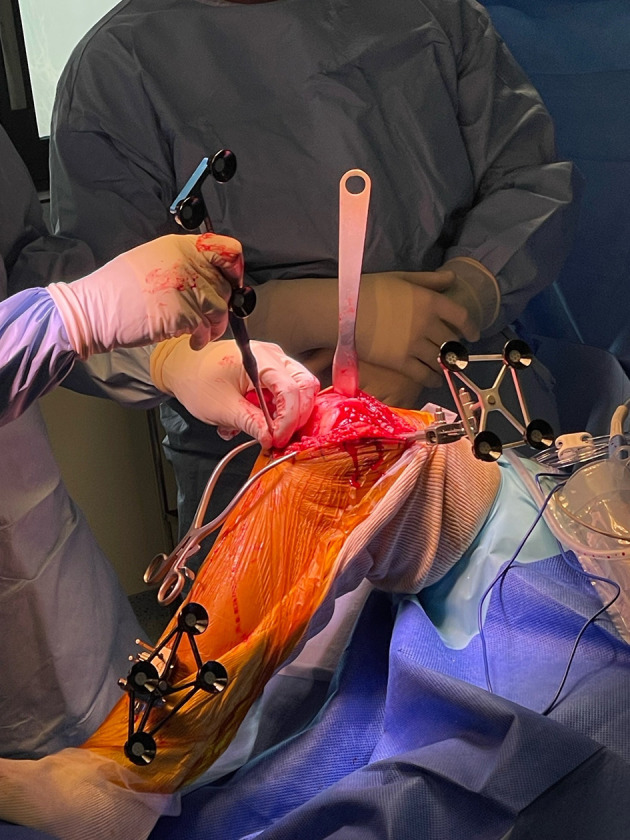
Arrays positioned. The reference points are registered to match the anatomy of the patient to the preoperative CT scan.
